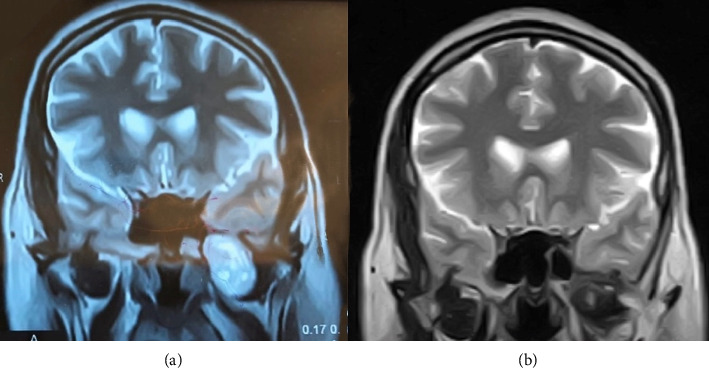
(a)Preoperative MRI shows a lesion measuring 50 × 40 × 20 mm in the left masticator space, extending superiorly up to the left temporal lobe. (b) Postoperative MRI done at 3 month interval showing complete removal of the tumor.
